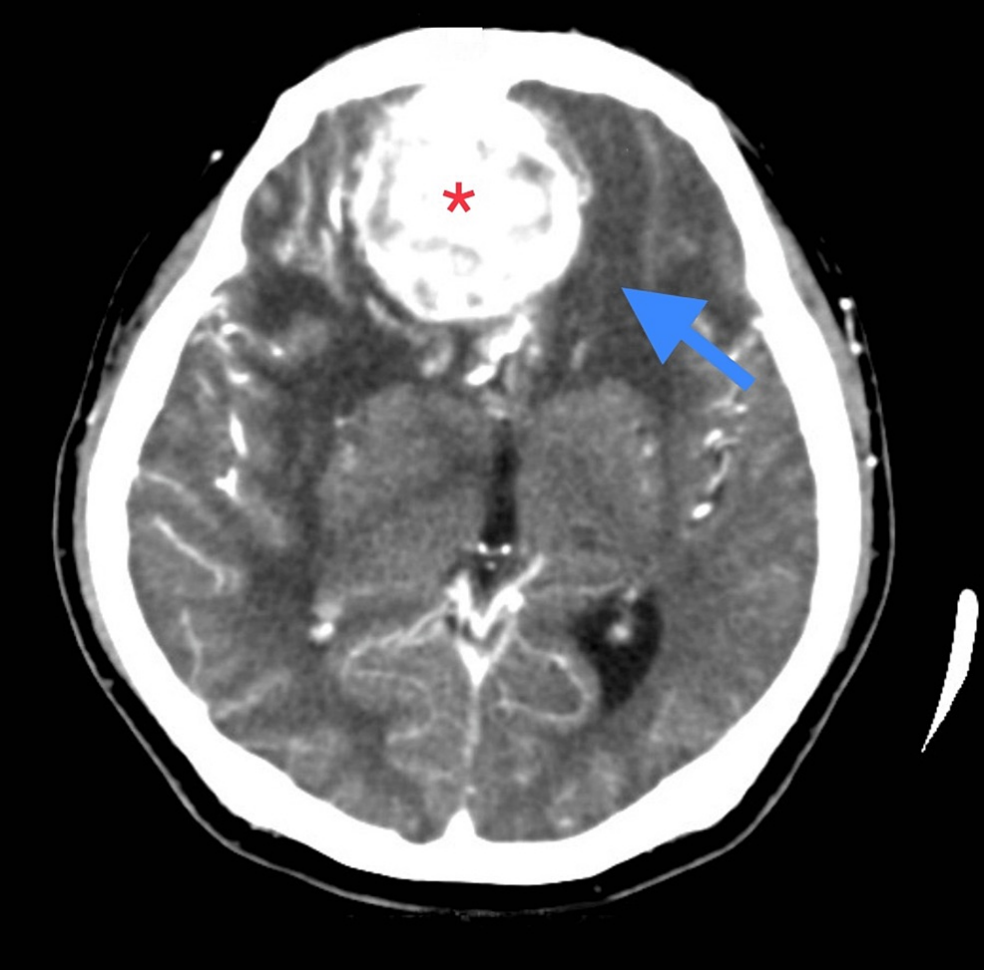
CECT Brain (axial view) showing bi-frontal extra-axial space-occupying lesion (red asterisk) measuring 4.8 x 5.0 x 4.8 cm with skull base erosion. There is a presence of perilesional oedema (blue arrow), left midline shift with ipsilateral ventricle compression. CECT: Contrast-enhanced computed tomography
